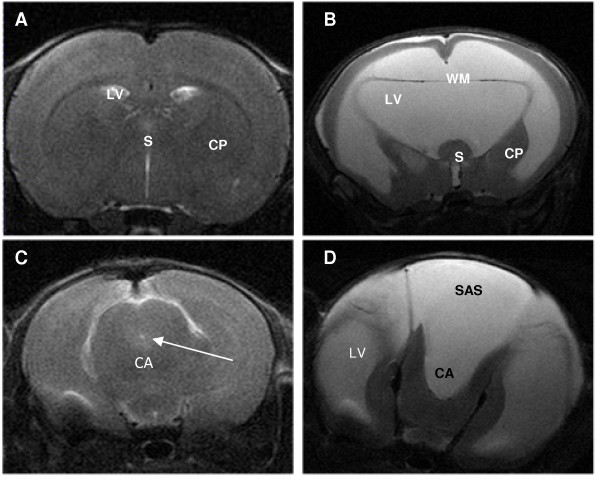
Representative coronal slices of T2-weighted MRI images comparing 21 day post-saline controls (A, C) to 21 day post-kaolin hydrocephalic brains (B, D). The hydrocephalic brains exhibited a dramatic increase in size of the lateral ventricles (LV), as well as the gross distortion of the caudate putamen (CP) and septum (S) following kaolin injections. The periventricular white matter (WM) appears to be thinned, stretched, and detached from the overlying cortical grey matter by either expansion of the lateral ventricle or edematous tissue. Additionally, image D demonstrates the cerebral aqueduct (CA) expanding caudally creating a possible communication to the subarachnoid space (SAS) that overlies the dorsal surface of the midbrain.
